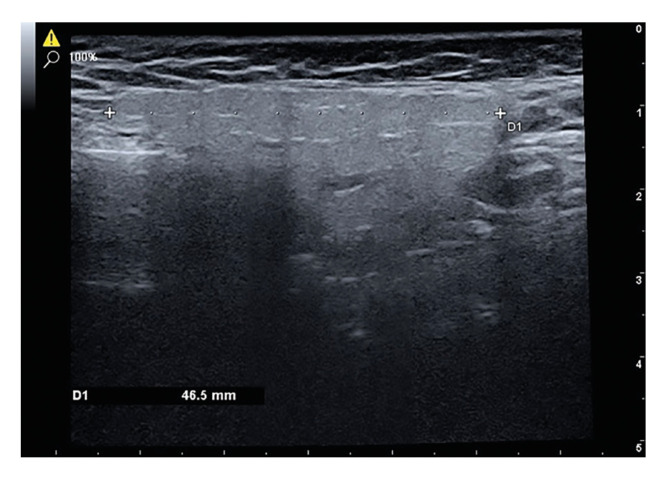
Superioinferior dimension measurement in a longitudinal ultrasonographic image of the parotid gland obtained using the panoramic scanning feature.
